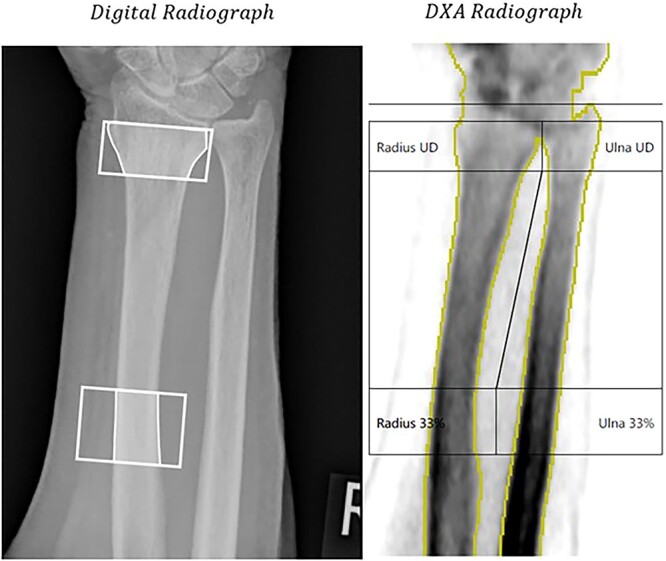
Left: Digital radiograph with ROIs shaded that are automatically produced using IBEX Bone Health. Right: Dual energy X-ray absorptiometry radiograph with ROIs after adjustment by reporting radiographer.
